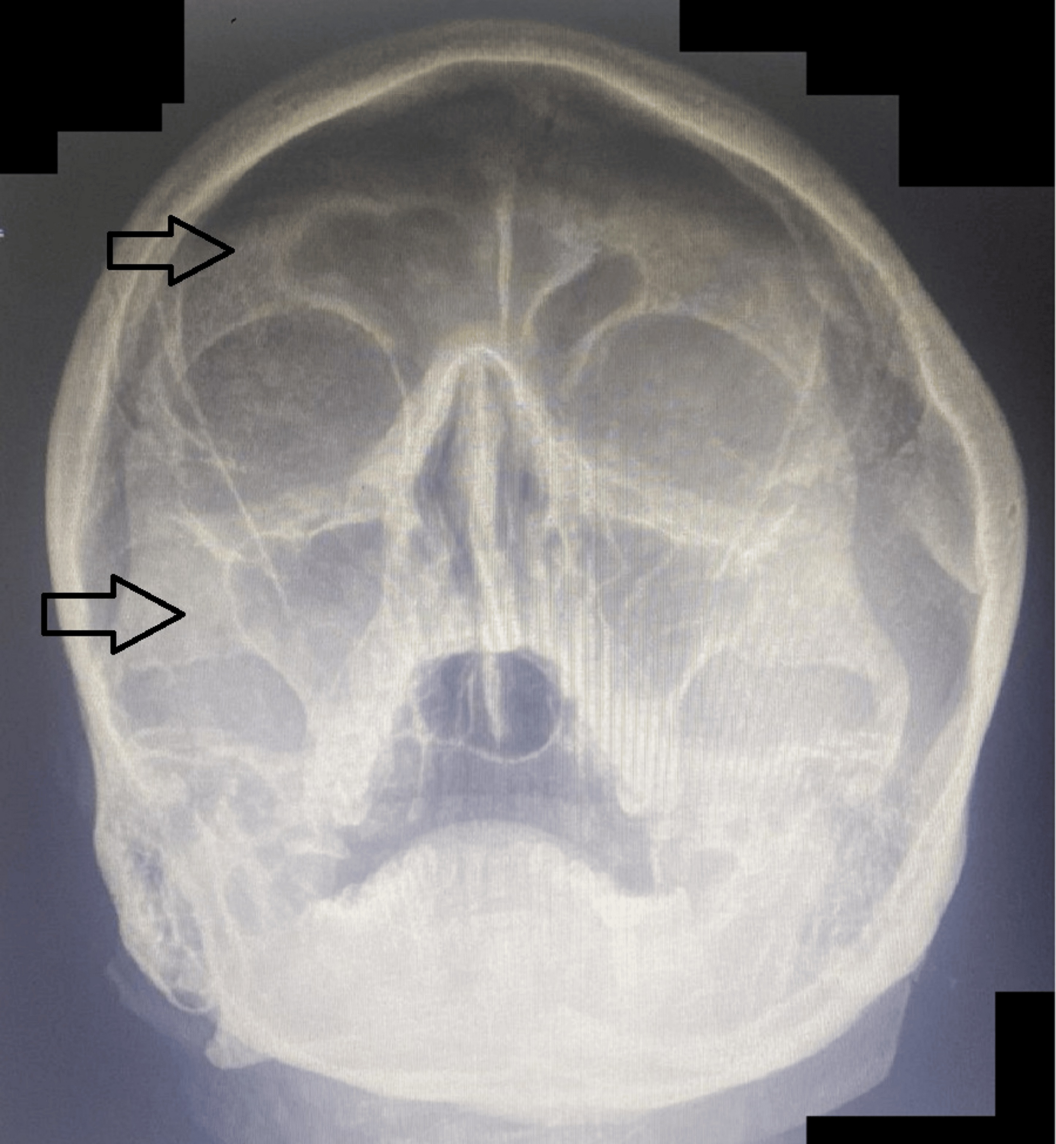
Radiograph of paranasal sinus showing bilateral maxillary sinus haziness and right frontal sinus haziness suggestive of sinusitis
